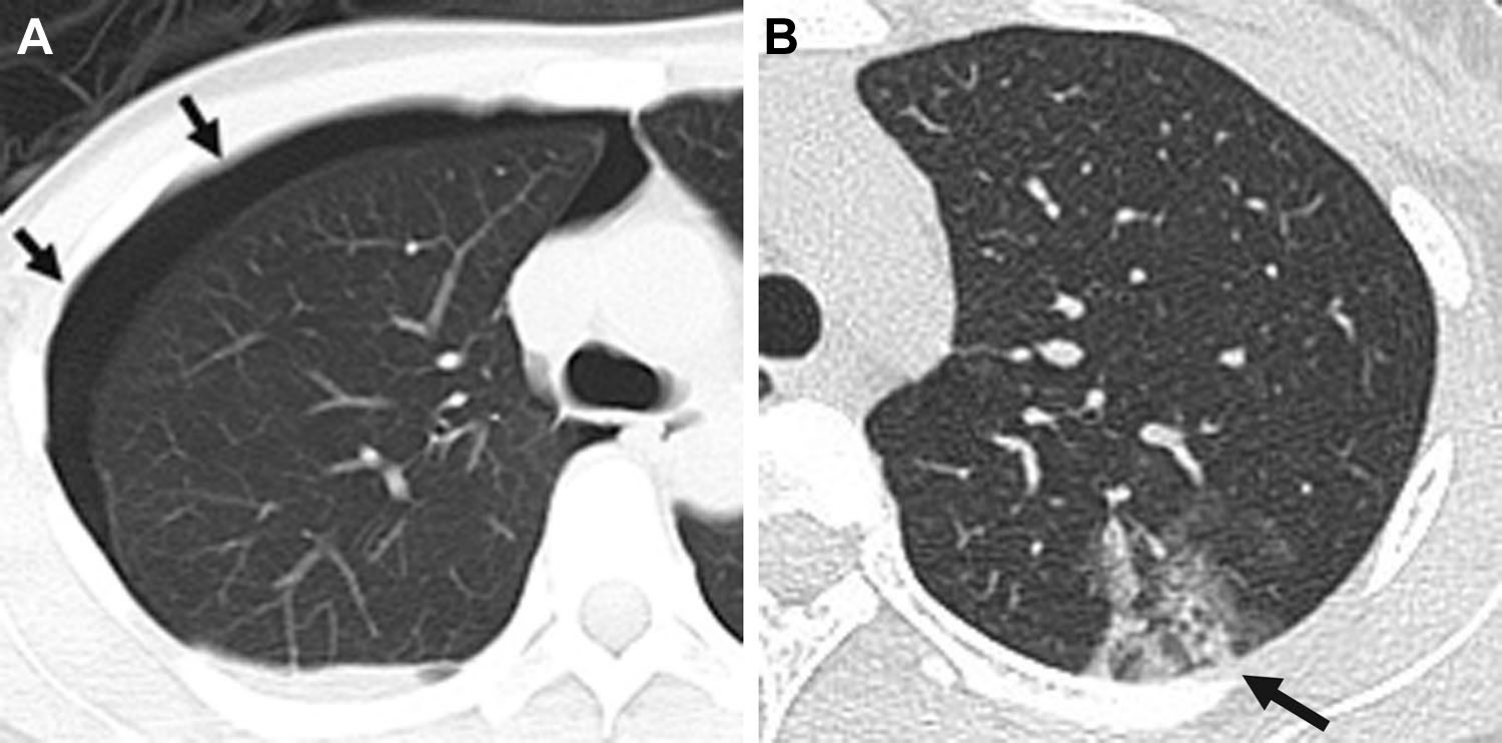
Thoracic endometriosis. A 36-year-old with repeated catamenial right pneumothorax. A On CT, right pneumothorax (arrows) is observed. A 28-year-old with repeated catamenial hemoptosis. B On CT, patchy ground-glass opacity (arrow) at the left upper lobe reflecting pulmonary hemorrhage is observed
